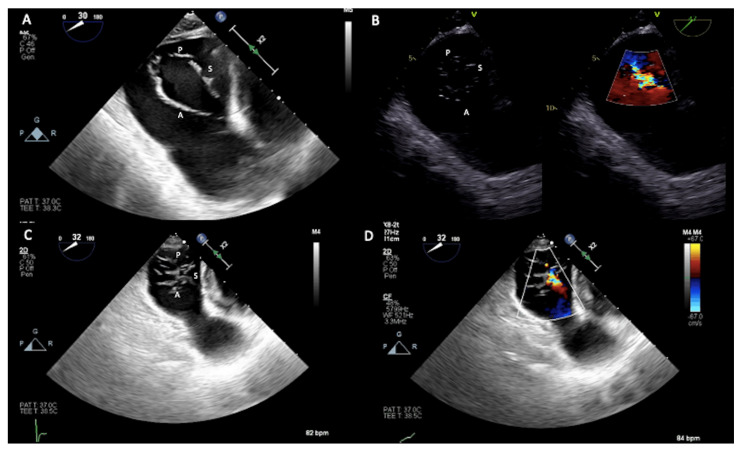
Two-dimensional transesophageal echocardiogram transgastric (TG) views. (A) TG short axis 30° documenting all three tricuspid valve leaflets in the open position during diastole; (B) in another patient, TG short axis 47° also with Color Doppler that documented functional tricuspid regurgitation due to leaflets tethering; (C) in another patient, TG short axis 32° also with color Doppler (D) with closed leaflets during systole documenting malcoaptation, especially between the anterior and septal leaflets, determining tricuspid regurgitation. The anterior leaflet (A) is in the far field, the posterior leaflet (P) is in the near field, and the septal leaflet (S) is on the right.
